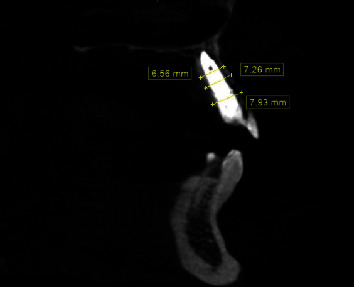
Computed tomography after 5 years of follow-up. It is noted that the buccal and palatal walls were maintained after expansion.
